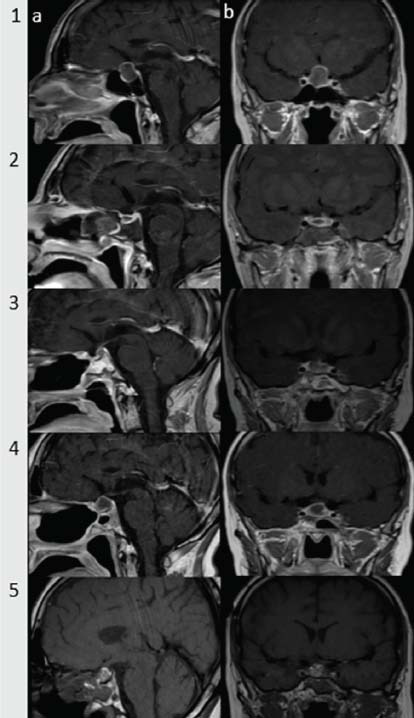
Preoperative sella MRI, sagittal and coronal plane. (a) T1-weighted hyperintense lesion with peripheral rim-like contrast enhancement was observed in the sella cavity. It measured approximately 2 cm and filled the cavity, slightly elevating the chiasm and extending towards the suprasellar cistern. The lesion showed minimal protrusion of the sphenoid sinus and encircled the internal carotid artery at an angle of approximately 180º, extending into the left cavernous sinus. (b) MRI of early postoperative sella, sagittal and coronal plane. (c) MRI of the Sella in the third month after surgery, sagittal and coronal plane. (d) MRI of the Sella in the first postoperative year, sagittal and coronal plane. (e) MRI of the second ETSS, early postoperative, sagittal, and coronal plane.
